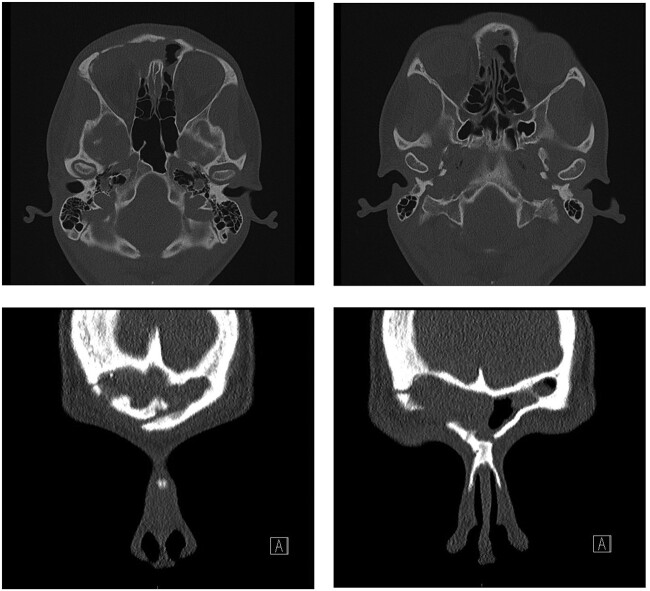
Repeat CT scan at 4 months after surgery, demonstrating no residual disease and radiological improvement of proptosis.
